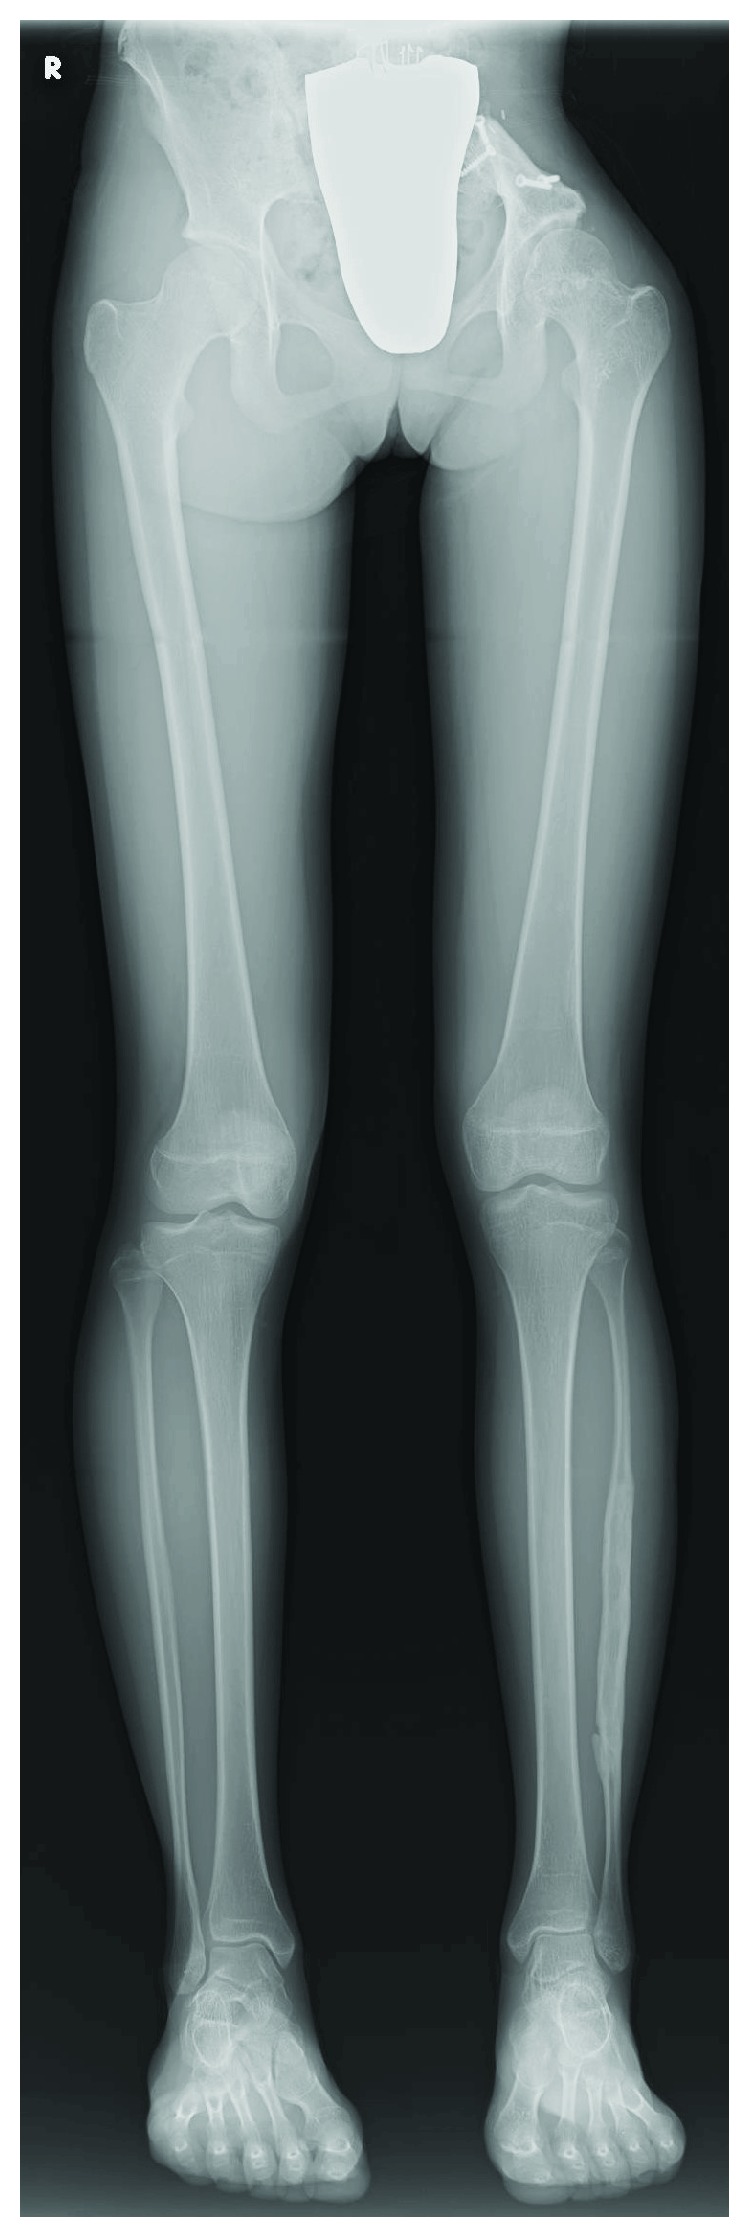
Plain radiograph of the patient's whole legs in a standing position at 5 years 10 months showing regeneration of the left fibula.
